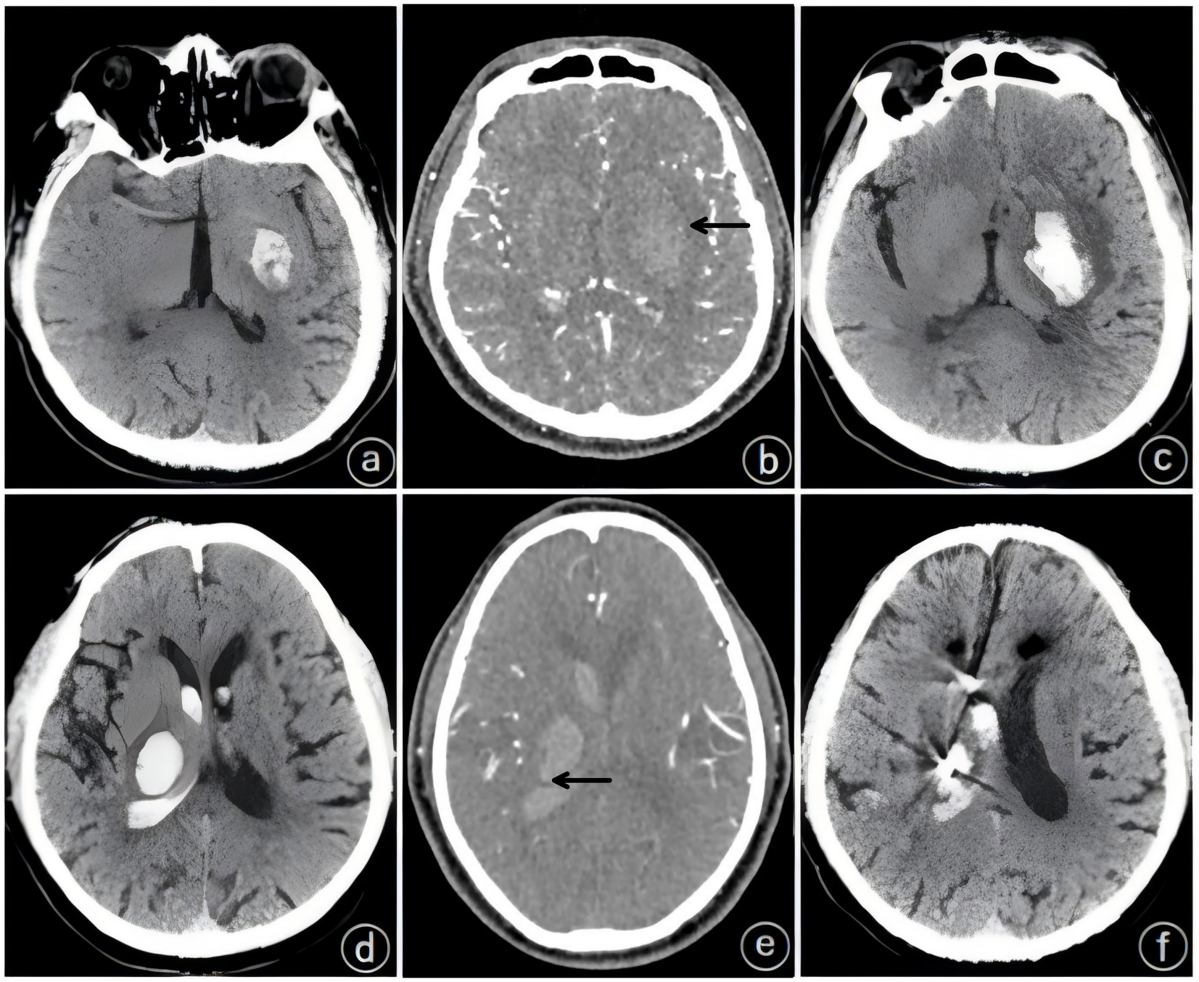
Typical cases of a single spot sign (black arrow). (a–c) Patient: male, 58 years old, a: initial hematoma of 15 mL, (b) spot sign seen on computed tomographic angiography (CTA) within 24 h, (c) hematoma expansion (HE) on preoperative repeat computed tomography (CT). (d) Patient: male, 74 years old, initial hematoma of 23 mL; (e) spot sign on computed tomographic angiography (CTA) within 24 h; (f) no hematoma expansion (HE) on postoperative repeat computed tomography (CT).
